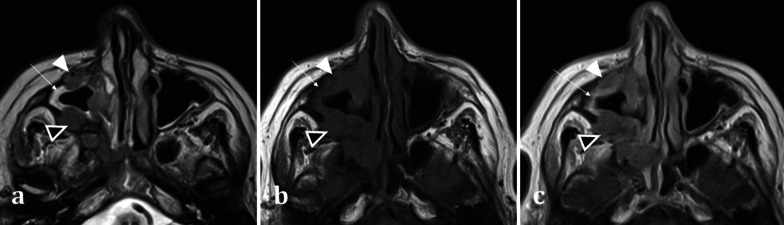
Axial MRI images of a 66-year-old female patient with right maxillary sinus squamous cell carcinoma (SCC). She presented with symptoms of chronic rhinosinusitis for which a CT sinuses was performed (not shown) demonstrating unilateral maxillary sinus disease, suspicious for malignancy. Axial T2 weighted image (a) exhibits an isointense soft tissue lesion (white arrowhead) permeating and extending through the right maxillary sinus, which can be differentiated from the high T2 signal normal mucosa (arrow). Both the SCC (white arrowhead) and normal mucosa (arrow) are hypointense on T1W imaging (b). On T1W post-contrast imaging (c), the lesion demonstrates less enhancement (white arrowhead) compared to the adjacent hyperenhancing sinonasal mucosa (arrow) (c). Transpatial spread into the right pterygopalatine fossa is also identifiable on all sequences (black arrowheads with white outline)
